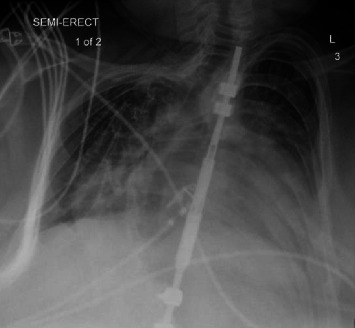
Chest X-ray during Pediatric Intensive Care Unit admission demonstrating enlarged cardiac silhouette, nonspecific bilateral airspace opacities, perihilar interstitial markings, and haziness of the left costophrenic angle. No evidence of major pleural effusion or pneumothorax. Thoracic scoliosis and posterior spinal growing rod are visualized. Suboptimal evaluation related to body habitus.
